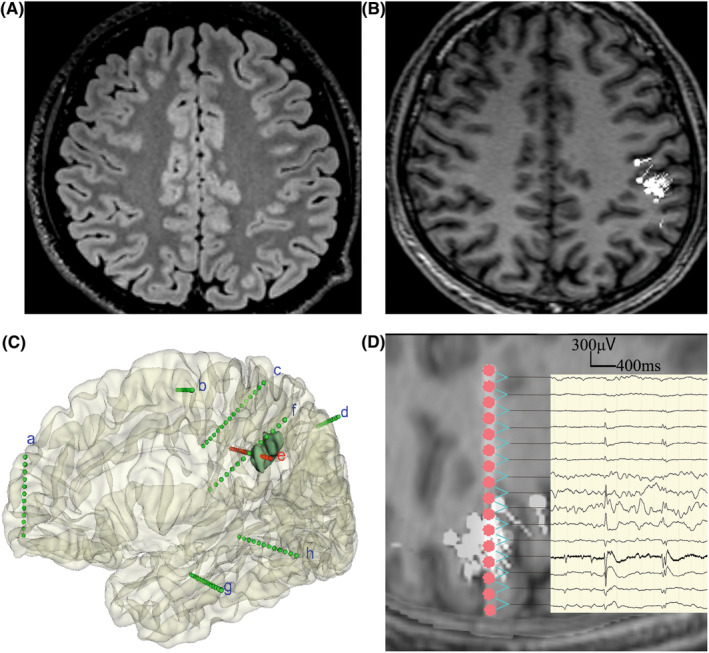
MEG navigate resection in a patient with negative MRI findings. A male patient, 22 years old, with a 7‐year history of epilepsy. This patient had a complete resection of MEG dipole cluster. At the 12‐month follow‐up visit, this patient achieved a favorable clinical outcome (Engel Class I). (A) The result of MRI in fluid‐attenuated inversion recovery sequences; (B) the results of MEG: a single cluster in the supramarginal gyrus; (C) 3D reconstructions of participants' brains and SEEG electrode contacts, the green part is the resection lesion, the electrode contacts are shown by pink or greed points; (D) a combination of the MEG‐electrode‐SEEG wave, the electrode contacts are shown by pink points, while the intracranial EEG waveforms are shown in bipolar montages beside the contacts, in one‐to‐one correspondence, the MEGSSs are shown in white points with small tails.
